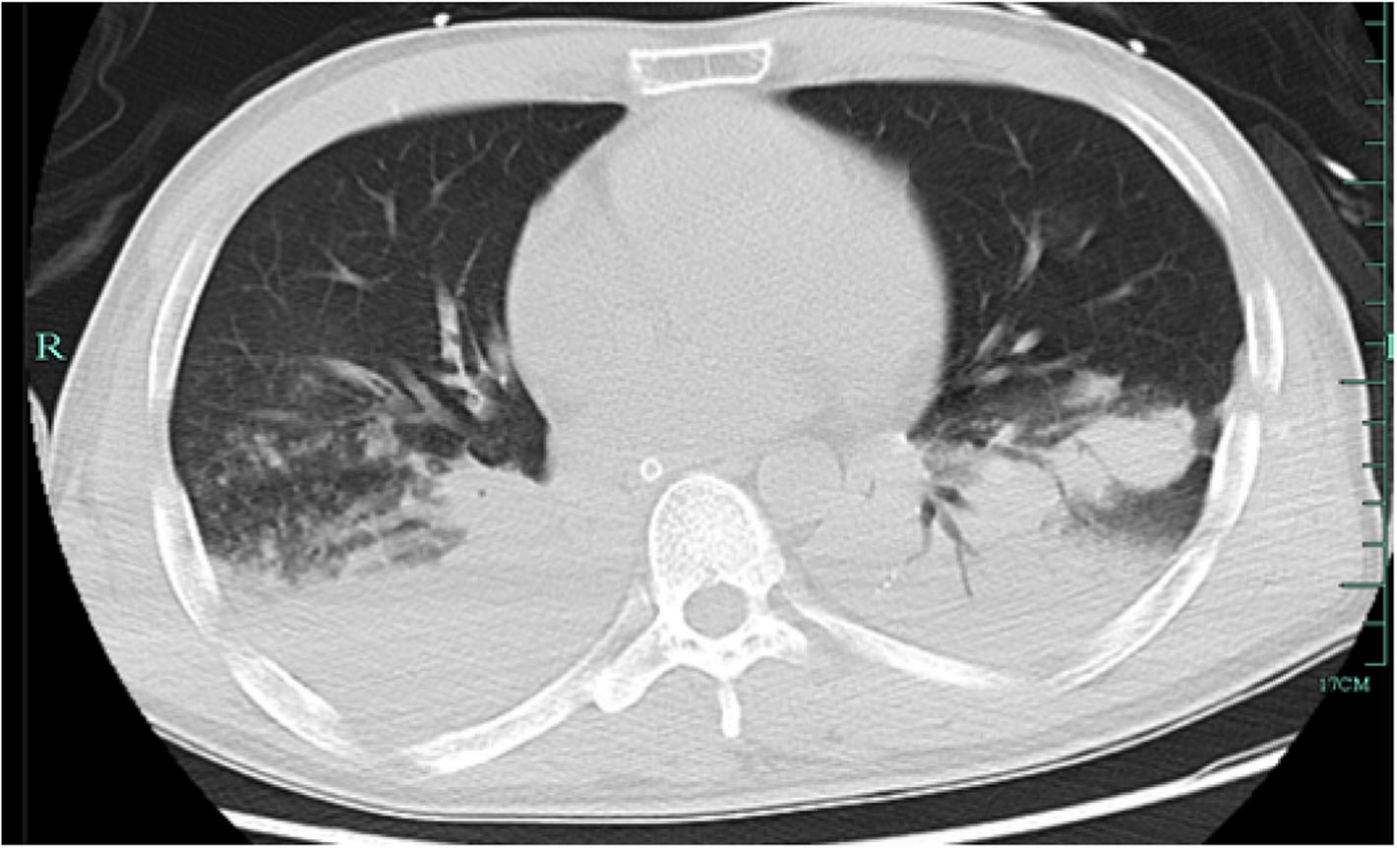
Lung CT scan on January 4, 2019. Lung CT taken on day 11 shows patchy consolidation in both lungs
